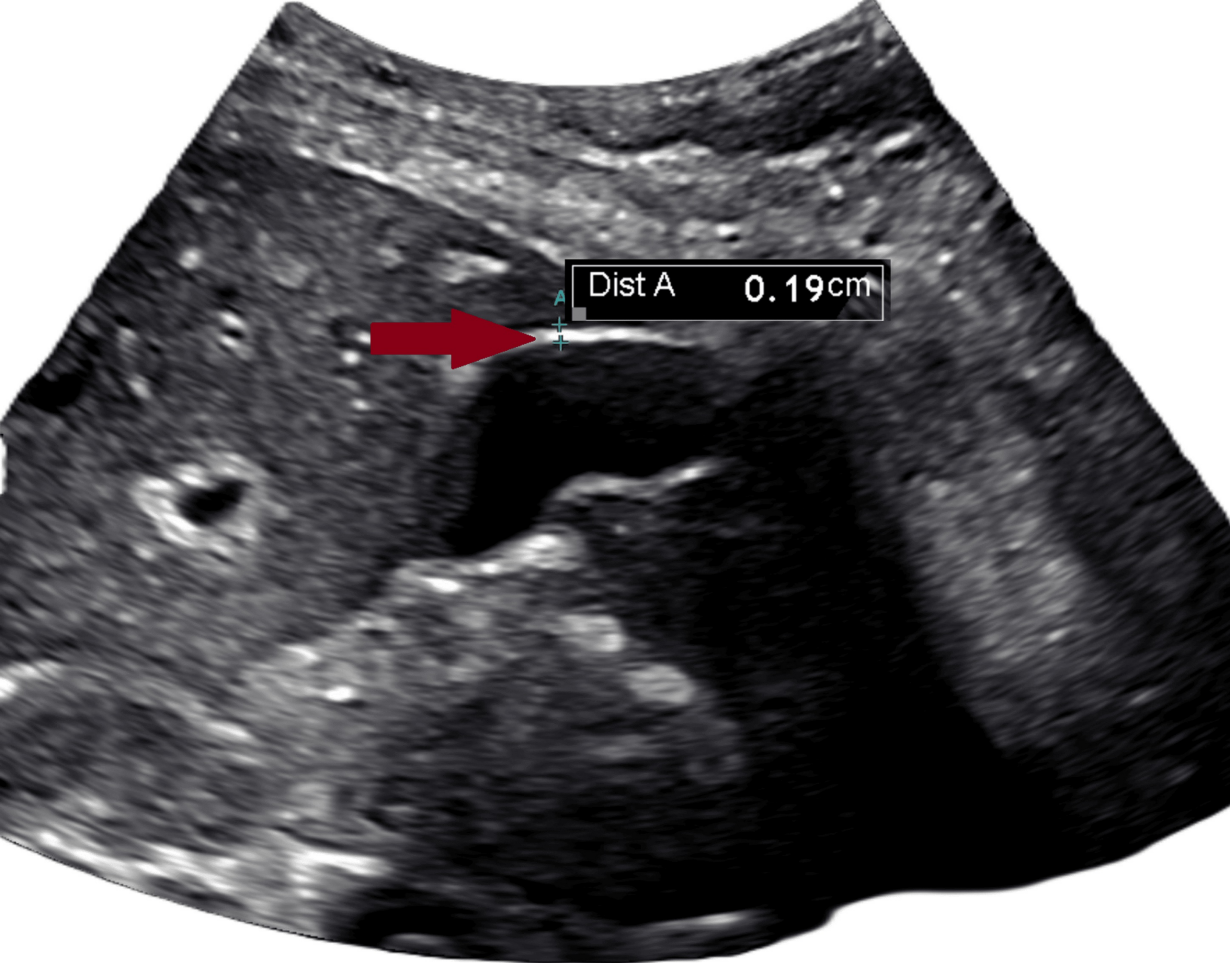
Right upper quadrant ultrasound image of the gallbladder The right upper quadrant ultrasound image (RUQ US) shows a normal gallbladder wall (red arrow), measuring 1.9 mm without thickening.
